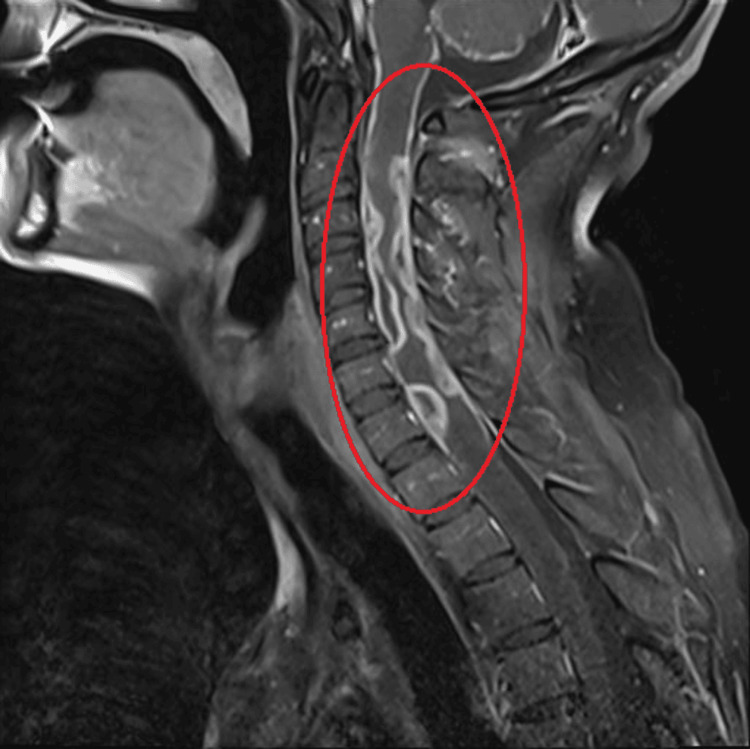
Magnetic resonance imaging of the cervical spine demonstrating diffuse cord edema and multiple rim-enhancing subdural abscesses
